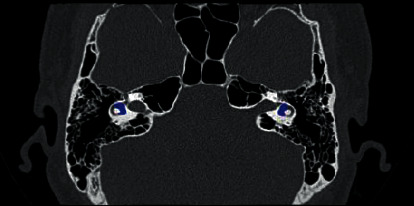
An example of the vestibule structure from CT image. The vestibule is highlighted in blue.
